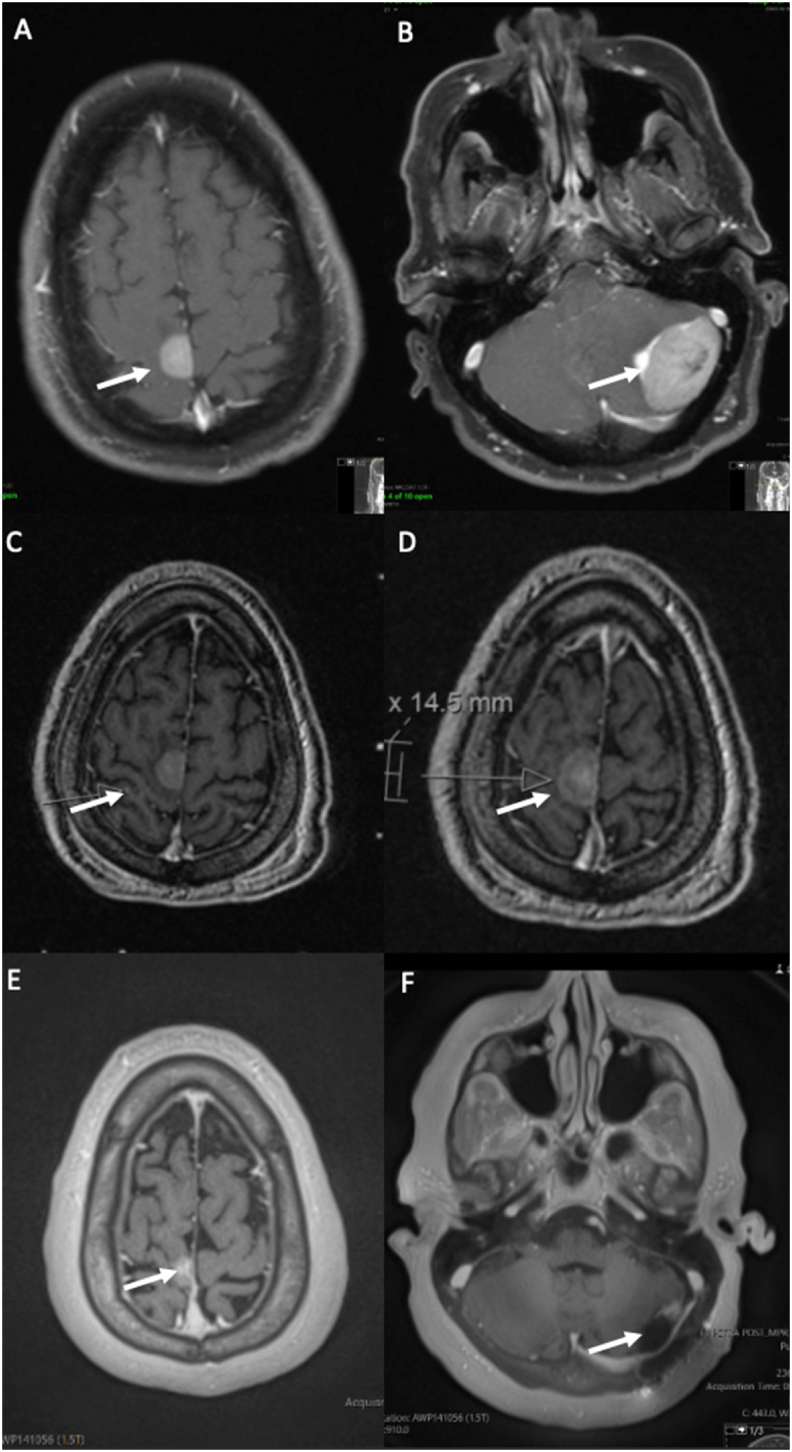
T1-weighted post-contrast brain MRI images. A) Lesion in right posterior frontal parasagittal cortex (arrow). B) Left cerebellar lesion (arrow) with marked vasogenic edema and compression of the fourth ventricle. C) Post-craniotomy imaging showing new punctate lesion (arrow). D) Post-craniotomy imaging showing increased size of right posterior frontal parasagittal cortex lesion (arrow). E) Post-craniotomy and radiotherapy imaging of right posterior frontal parasagittal cortex lesion site (arrow). F) Post-craniotomy and radiotherapy imaging of left cerebellar lesion site (arrow).
